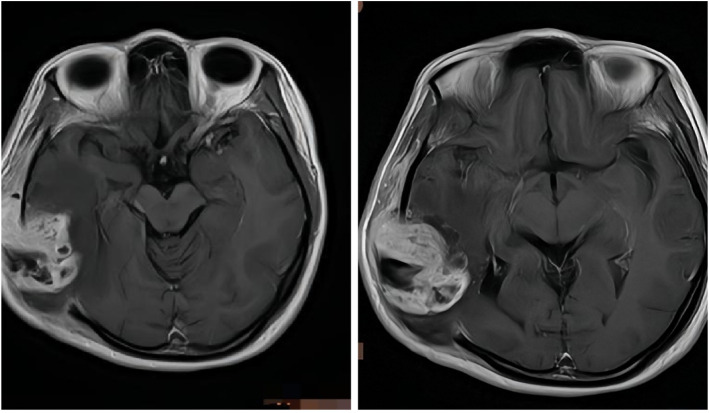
After surgery one month later, T1-weighted sagittal MRI showed that the solid component of the right temporal soft tissue mass was more enlarged than before.
